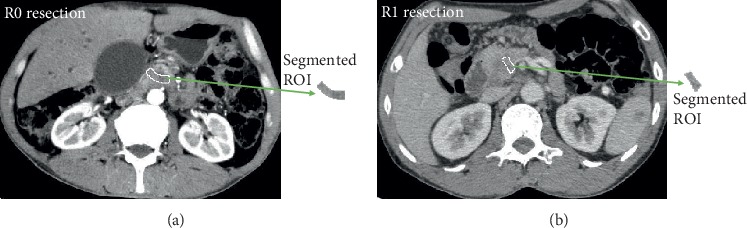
Delineate a resection margin and segment it to form an ROI. (a) A portal-venous phase CT image located at the top of a pancreatic head tumor that belongs to R0. (b) A portal-venous phase CT image located at the top of a pancreatic head tumor that belongs to R1.
